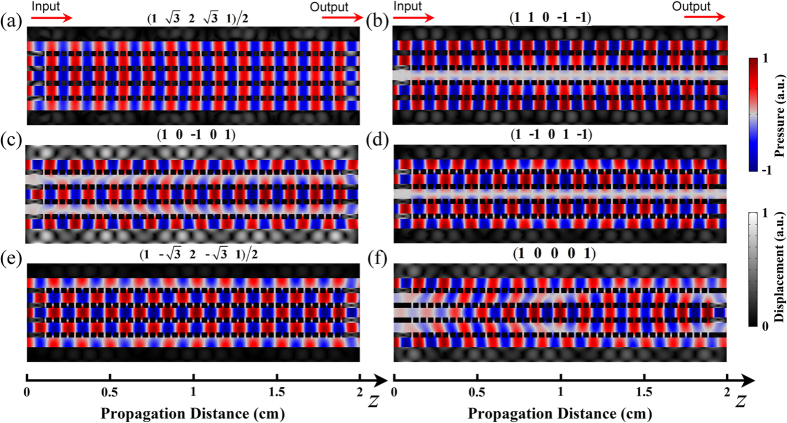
Pressure fields of different propagating modes in the ultrasound regime. (a–e) Normalized pressure field distributions of five different supermodes at *m* = 1, 2, 3, 4, 5, respectively. The weight factors of all water channels for each supermode are appended on top of the corresponding panels. (f) Normalized pressure field distribution when the input does not form a supermode. The operation frequency *f* = 1.02 MHz.
